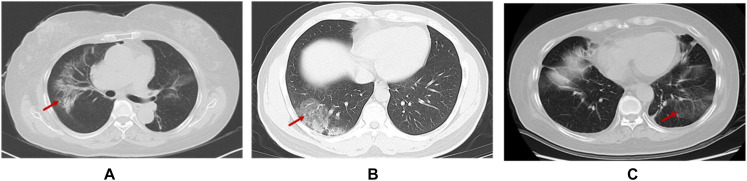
CT scan of patients contracted with ICI-pneumonitis (A) and viral pneumonitis [(B) (90) and (C)], respectively. (A) Female, 71 years old, advanced lung adenocarcinoma receiving immunotherapy for 8 months in our cancer hospital (C) Female, 62 years old, advanced lung adenocarcinoma, receiving chemotherapy in our cancer hospital.
